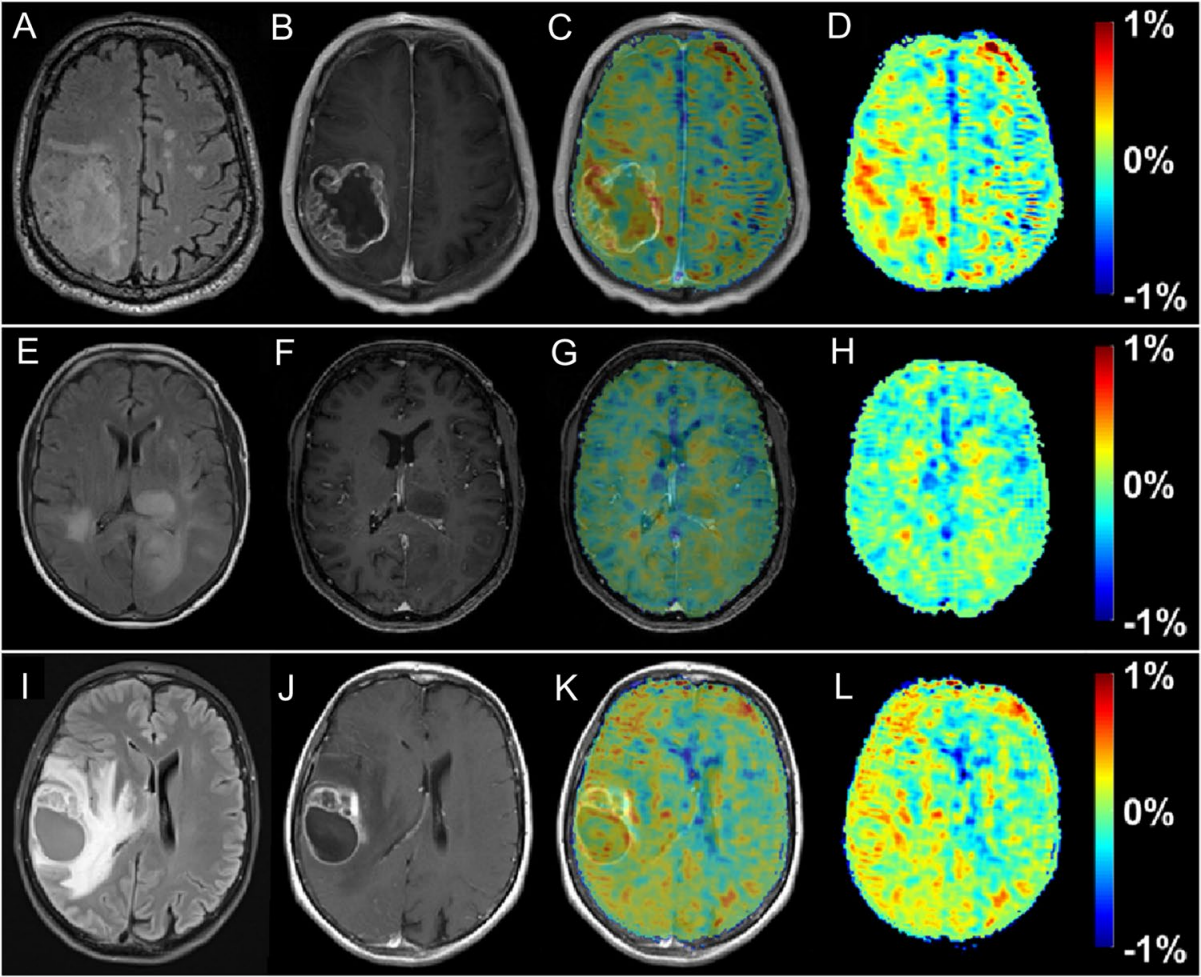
T_2_ FLAIR (A, E, I), T_1_‐ce (B,F,J), T_1_‐ce with overlaid ∆DGE_ρ_ map (C, G, K), and the ∆DGE_ρ_‐maps (D,H,L) of patient 1 (A–D, ~ 7 min post-injection), 2 (E–H, ~ 7 min post-injection), and 3 (I–L, ~ 9 min post-injection). From [22], with permission
